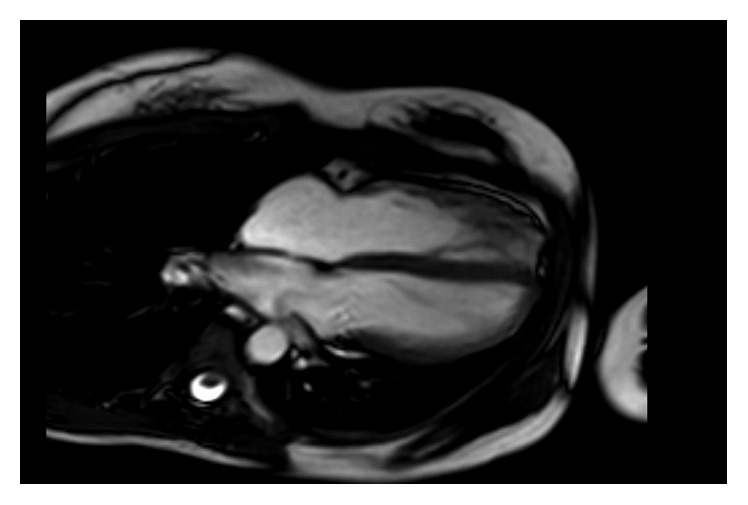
MRI cardiac showing inferoposterior position of the heart.
